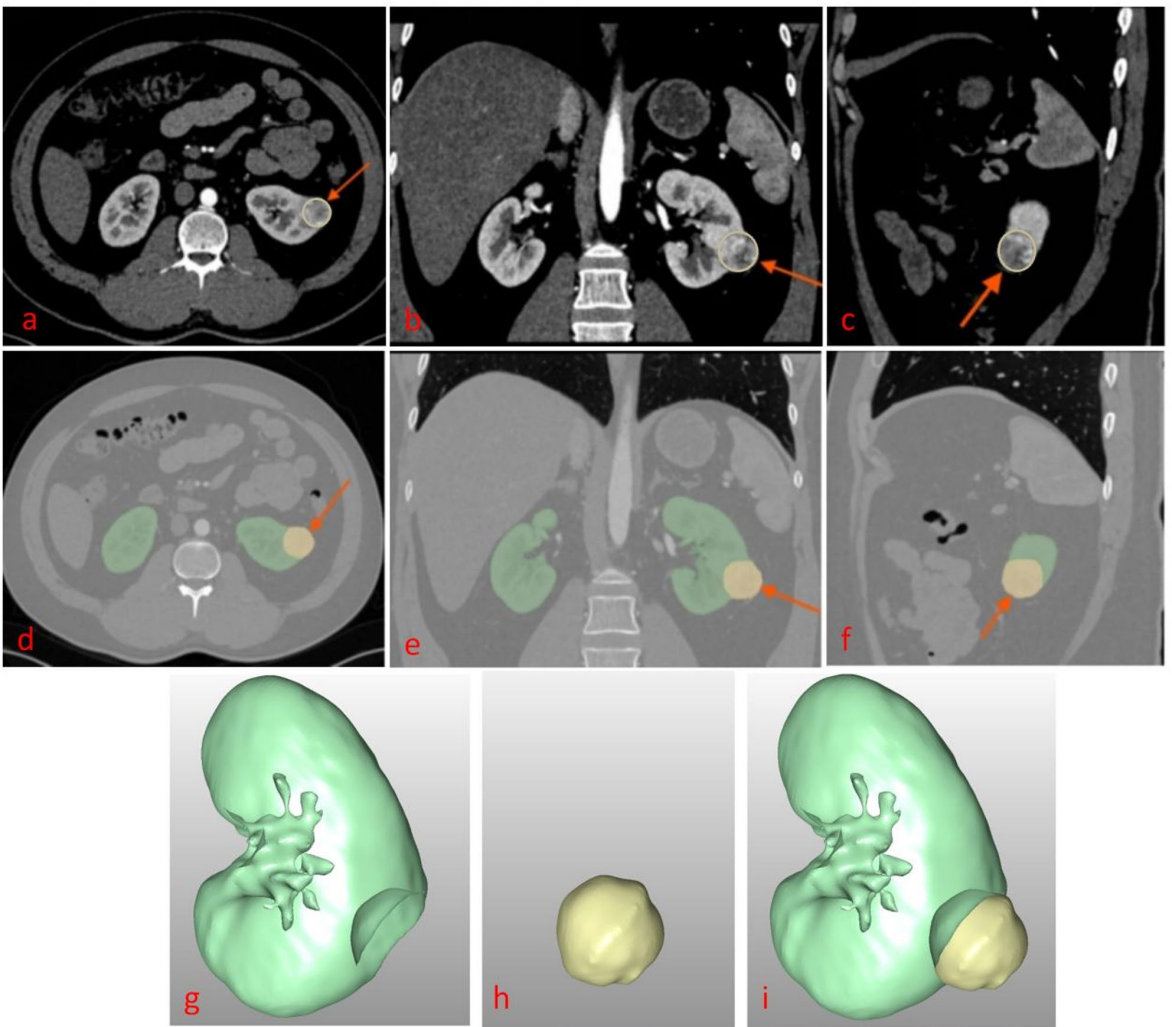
A 31-year-old male with incidentally detected left renal clear cell carcinoma (pathological stage T1a, grade 2). Panels a, b, and c show original axial, coronal, and sagittal CT arterial-phase images, respectively (red arrows: tumor). Panels d, e, and f depict corresponding axial, coronal, and sagittal views of AI-annotated kidney and tumor contours (red arrows: tumor). Panels g, h, and i present 3D reconstructed segmentation labels for the kidney, tumor, and their combined overlay, respectively
